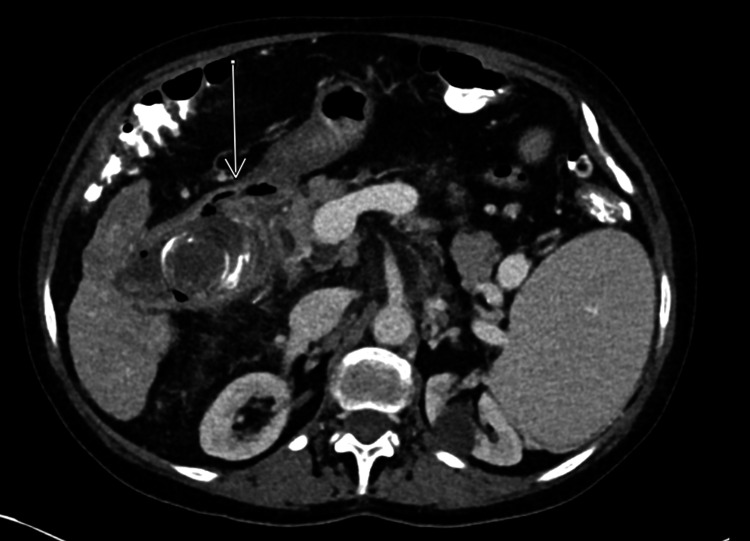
CT demonstrating fistulization of the gallbladder neck anteriorly into the duodenum (arrow) CT: computed tomography
